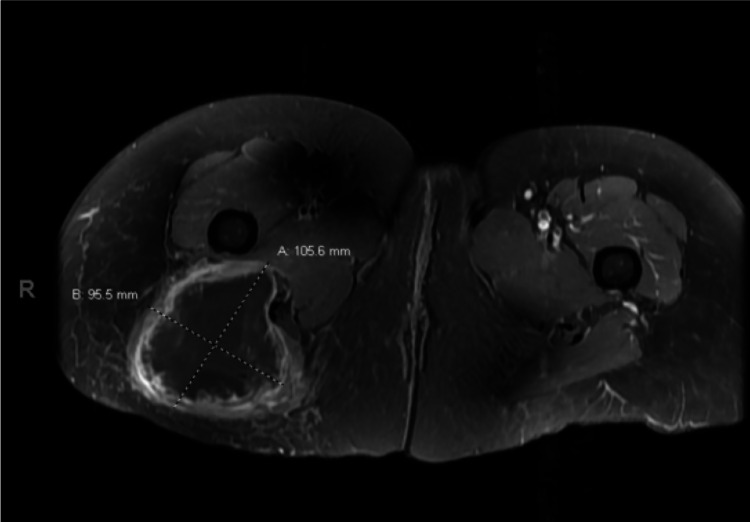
T1-weighted magnetic resonance imaging of the pelvis T1-weighted MRI of the pelvis demonstrates a round, predominantly hypointense lesion measuring 10 × 10 × 11 cm^3^ within the right gluteus maximus muscle. There is predominantly peripheral enhancement and internal T1 hyperintense debris/septation, suggesting central necrosis and internal hemorrhage.
